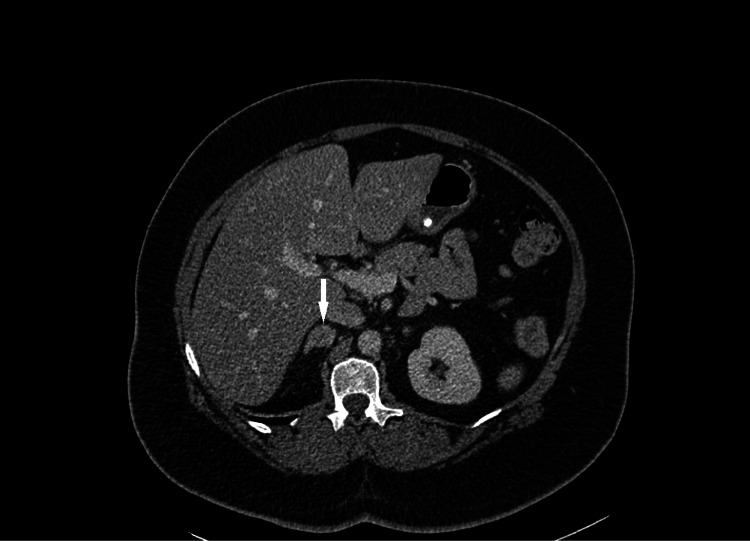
Computed tomography scan of the abdomen showing bilateral adrenal adenomas (right: 2 cm, left: 1.2 cm), with the arrow indicating the right adrenal adenoma
